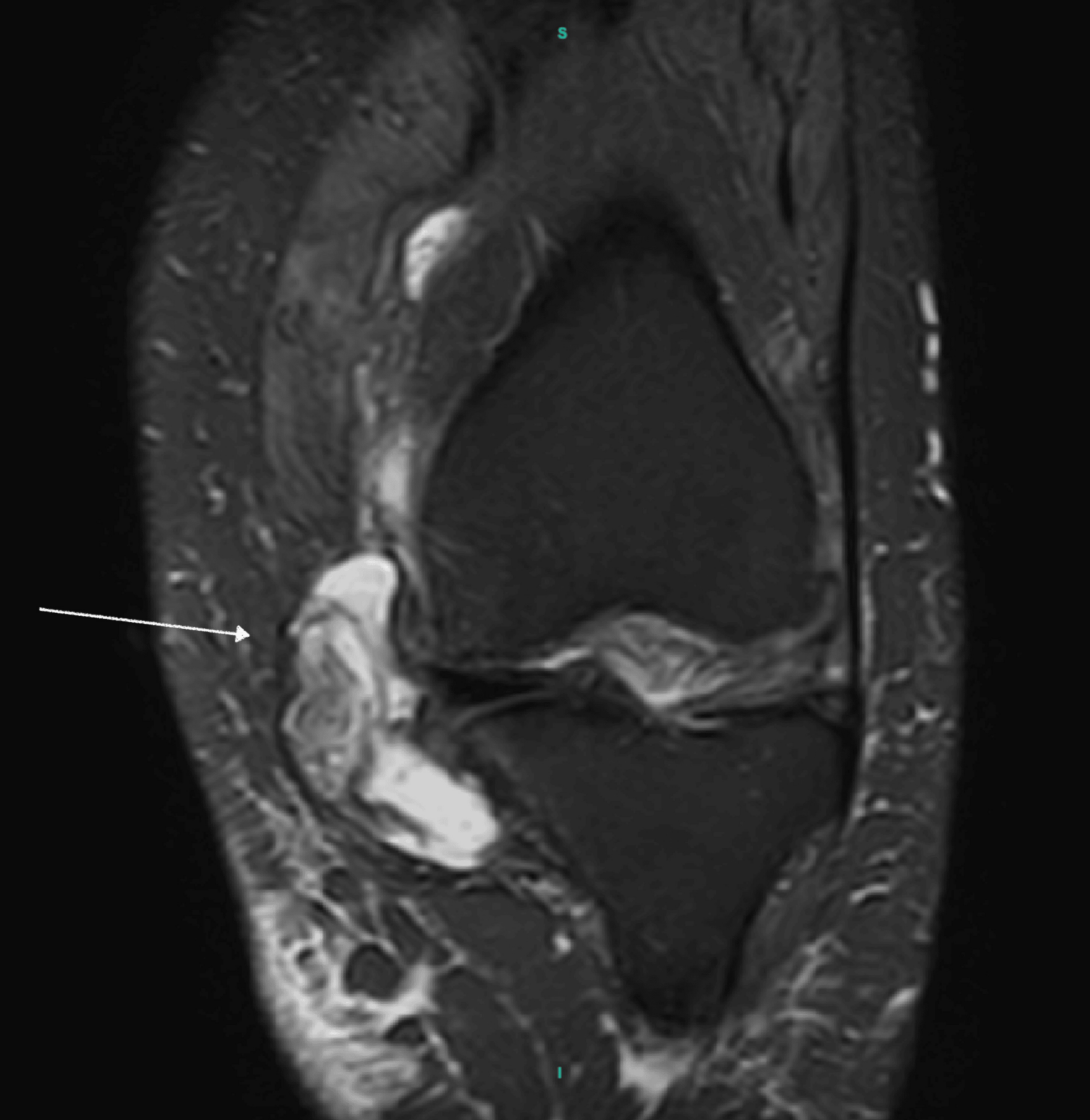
MRI demonstrating coronal view of the left knee showing the cyst having a mass effect on medial joint capsule (white arrow).
